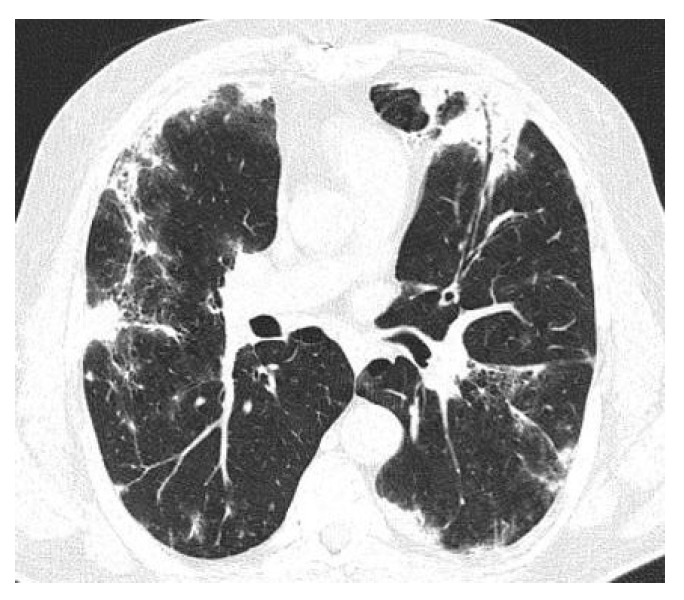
HRCT—July 2019—Case 1. Subpleural honeycombing, nodular lesions, linear fibrosis, traction bronchiectasis, reticulation and ground glass opacities with associated minimal emphysema. Diffuse pulmonary infiltrates in the inferior half.
